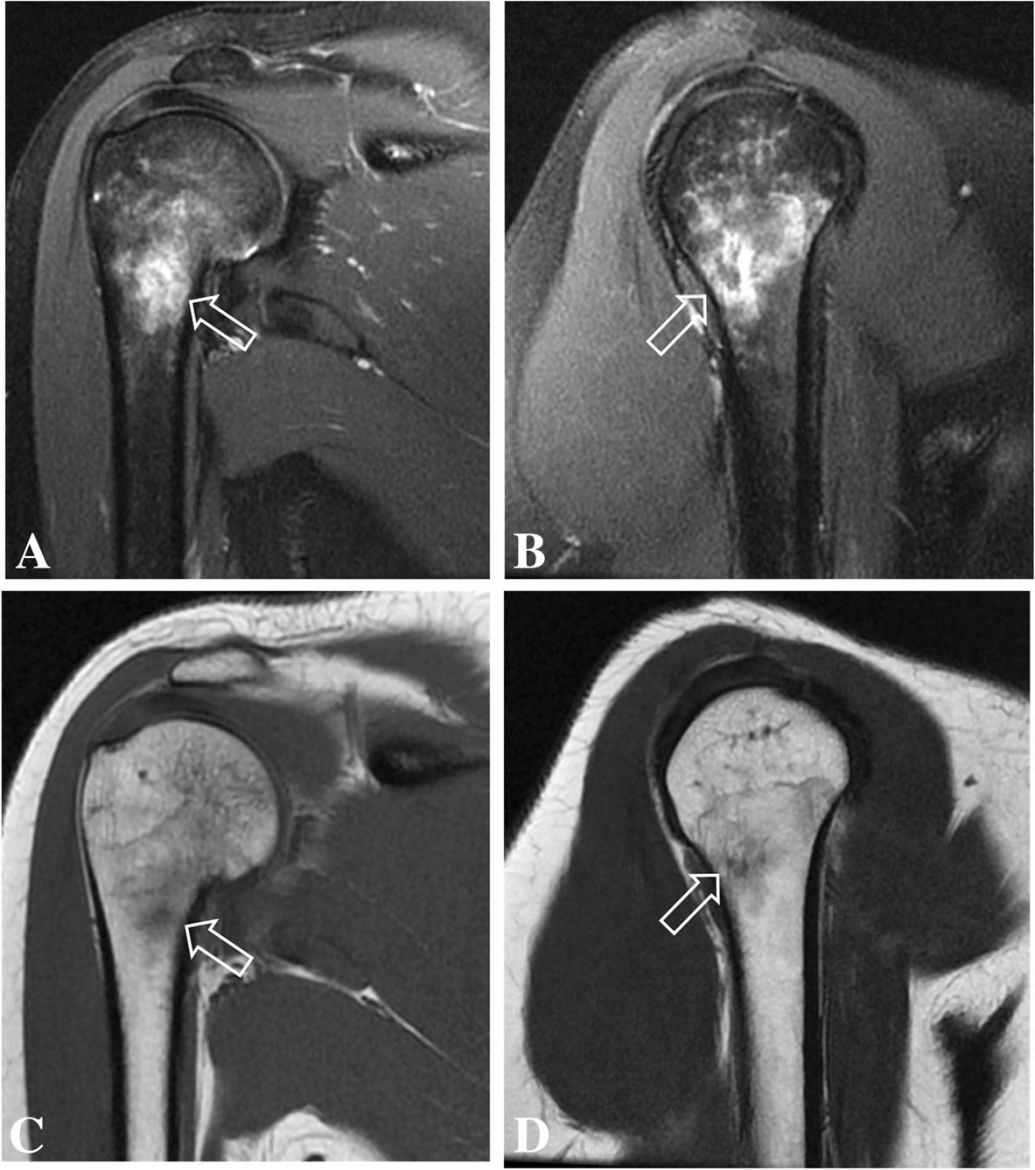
Coronal (a) and sagittal (b) T2-weighted fat-suppressed MR images and coronal (c) and sagittal (d) T1-weighted MR images of the right shoulder showing intense bone marrow edema of the proximal humerus, with a small incomplete transverse fracture line (open arrow)
